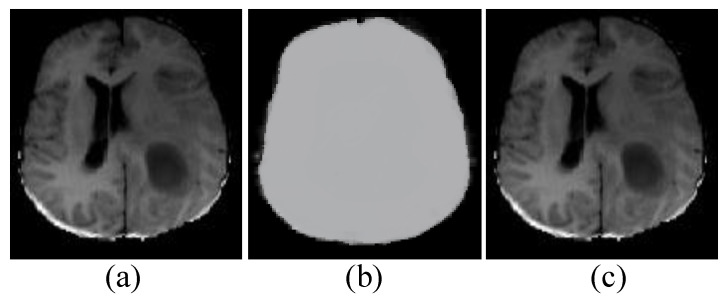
The impact of MR image intensity bias and the outcome of bias field correction. (a) before (b) the estimated bias field and (c) after the N4ITK bias field correction.
